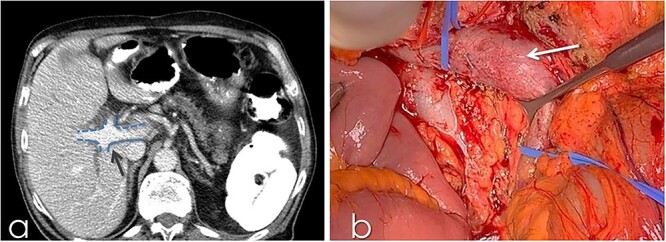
(a) CT with contrast demonstrating the trifurcated portal vein (arrow); (b) gross appearance of the duplicated IVC intraoperatively (arrow).
